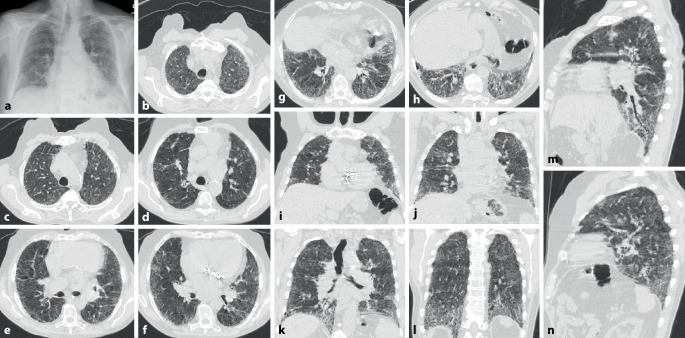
Die CT-Muster unterscheiden sich je nachdem, ob der Patient symptomatisch ist oder nicht.
